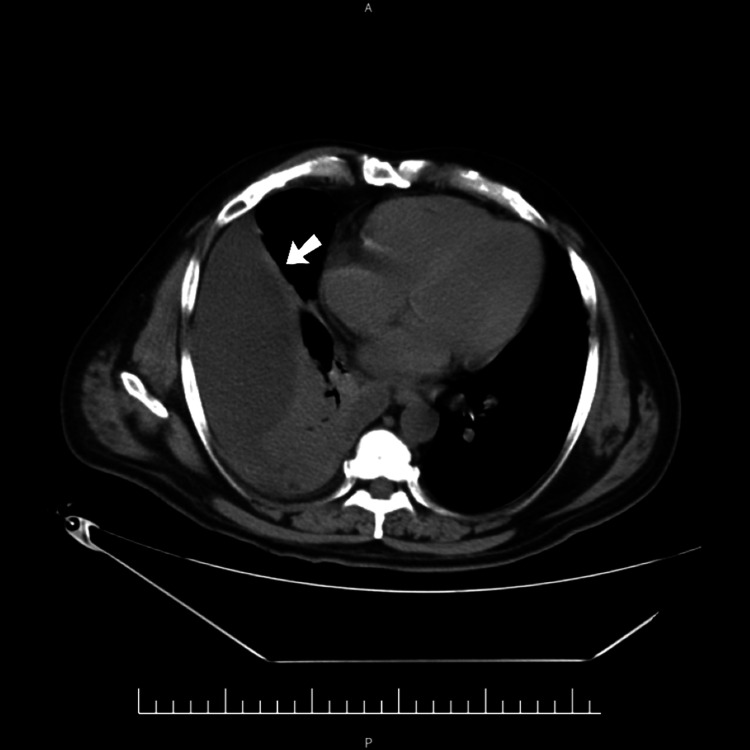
Computed tomography of the thorax. Contrast-enhanced computed tomography of the thorax (axial view) revealing a loculated, massive right pleural effusion (arrow). The fluid collection is causing complete collapse-consolidation of the right lower lobe and partial collapse of the middle and upper lobes.
